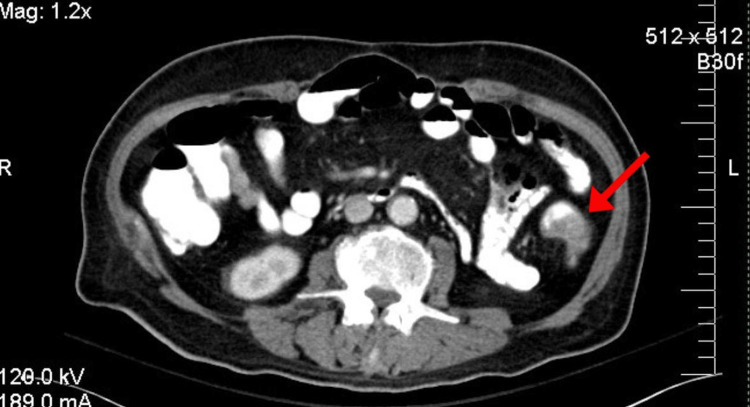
Axial view CT Abdomen with IV contrast. Mural circumferential thickening at the at inferior portion of the descending colon near the distal sigmoid 3.5 cm in length.
